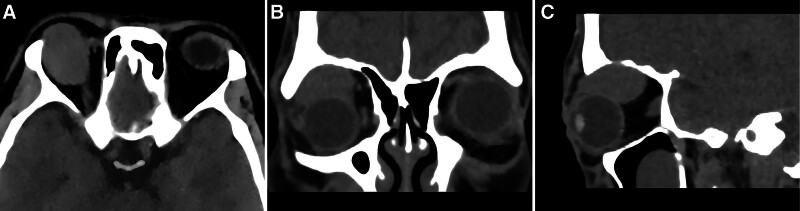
CT images. Transverse (A), Coronal (B), Sagittal (C). Suggested an oval soft tissue mass, unclear boundary with the anterior part of the superior rectus muscle, approximately 3.1 × 2.3 × 2.4 cm in size. CT = computed tomography.
